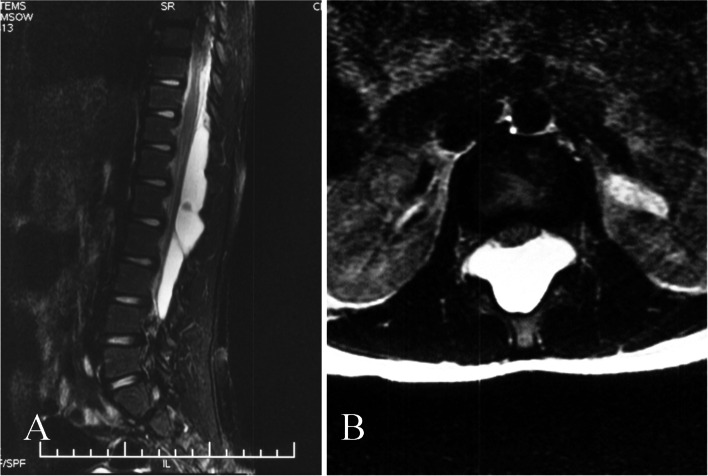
Flow void on MRI. A 7-year-old boy had weakness of the lower extremities, with dysuria, for 3 days. A Preoperative sagittal MRI showed a flow void with a SEMC at L1 level. B No flow void was found in axial MRI
